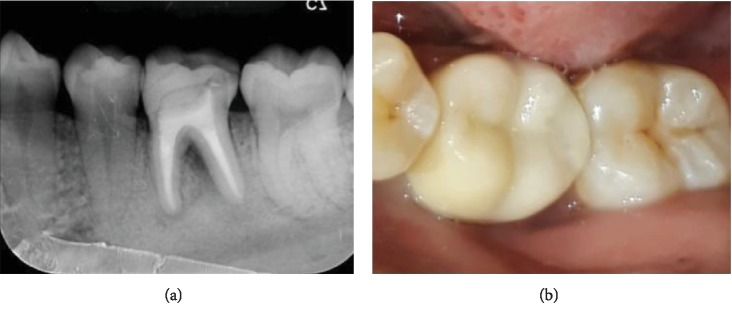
One-month postoperative evaluation: (a) postoperative radiograph; (b) Ceramage® onlay for restoration.
